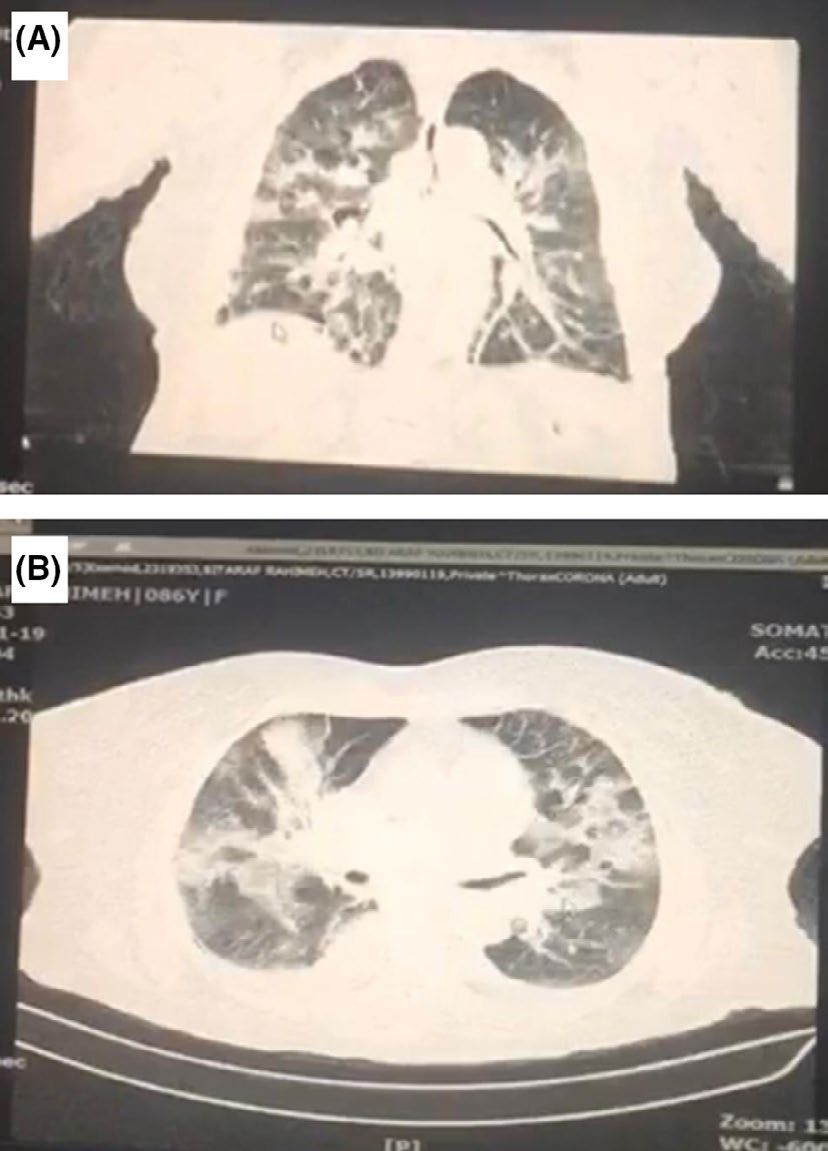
(A&B) Chest CT scan revealed bilateral consolidation
